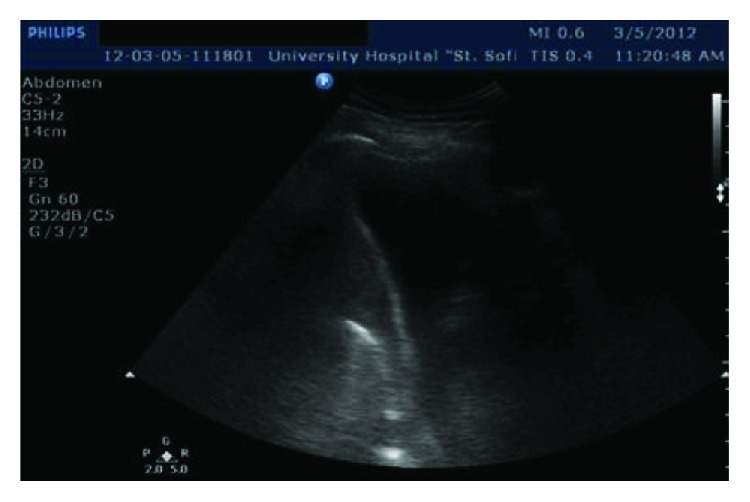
Ultrasound examination of the chest—a pleural effusion with a liquid and a solid (axillary and interlobar) component, evaluated as a hematoma, confirmed in the following surgical revision.
